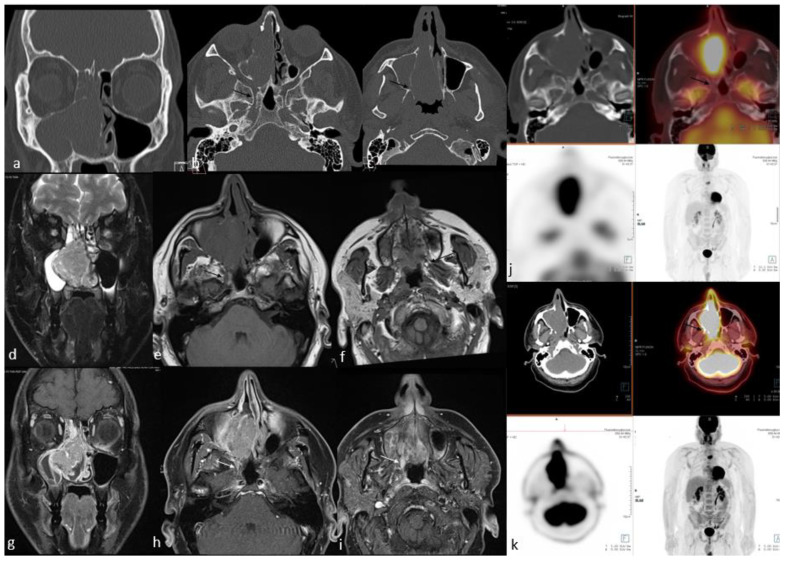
Sinonasal undifferentiated carcinoma. A 42-year-old male patient presenting with progressive symptoms of postnasal drainage, nasal congestion, and facial pressure. Initial CT (a) shows an expansile right nasal cavity lesion with medial bowing of the nasal septum and lateral bowing of the maxillary sinus wall. There is soft tissue density opacification in the right maxillary sinus; however, it is not possible to discern whether this represents postobstructive opacification or lesion extension. Coronal fat-saturated T2w (d) and post-contrast T1w (g) images better delineate the lesion and postobstructive mucosal opacification. Axial CT image (b) at the level of the vidian canal shows asymmetric enlargement of the right vidian canal (arrow, (b)) with asymmetric soft tissue intensity on the non-fat-saturated axial T1w (e) image and asymmetric enhancement on the post-contrast image (h,i). The ^18^F-FDG PET/CT image (j) at the same level shows FDG avidity of the primary nasal cavity lesion without uptake at the right vidian canal. More caudal axial CT (c) shows erosive changes in the right pterygoid plates with erosion of the greater palatine canal (arrow, (c)). There is loss of normal fat planes in the right greater palatine canal on the axial T1w image (f), with preservation of fat in the contralateral palatine canal (arrow, (g)). The postcontrast axial image shows asymmetric enhancement in the right greater palatine canal (arrow, (h)). ^18^F-FDG PET/CT image (k) at the same level shows FDG uptake extending into the right greater palatine canal. Pathology was consistent with high-grade carcinoma, most consistent with sinonasal undifferentiated carcinoma.
